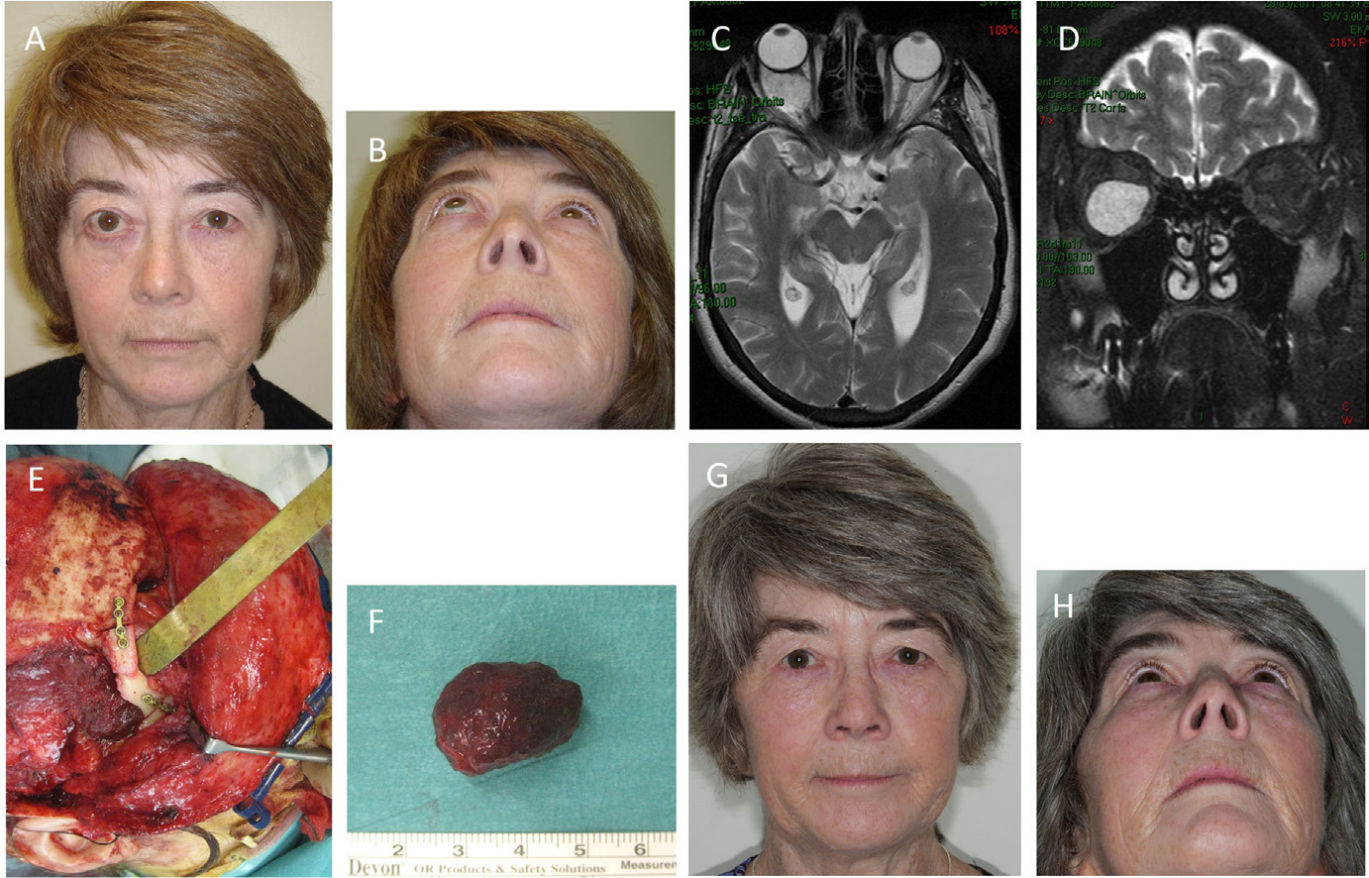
A 62-year-old female with right eye proptosis resulting from an intraconal venous malformation (VM) (A,B). Axial (C) and coronal (D) T2-weighted magnetic resonance imaging showing a 2.8 cm × 2.4 cm VM within the right orbital cone. The lesion (E) was removed through a lateral orbitotomy *via* a coronal incision. The orbitotomy was plated (E). Photograph of the lesion following removal (F). The position of the right globe was restored and remained satisfactory with no visual dysfunction 5 years postoperatively (G,H).
